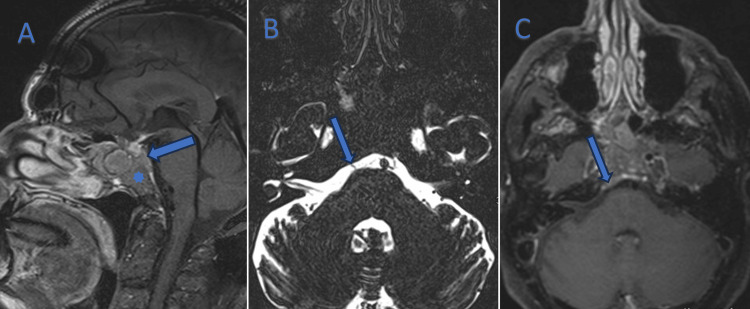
Brain MRI Sagittal dark blood contrast-enhanced (A): clival involvement (star). Notice the meningeal pre-clival tumor deposit (arrow). Axial space T2WI (B): right cisternal portion of the abducens nerve before entering Dorello’s canal (arrow). Axial dark blood contrast-enhanced (C): meningeal pre-clival tumor deposit (arrow) at the same level entry of the right abducens nerve in Dorello’s canal (see B).
